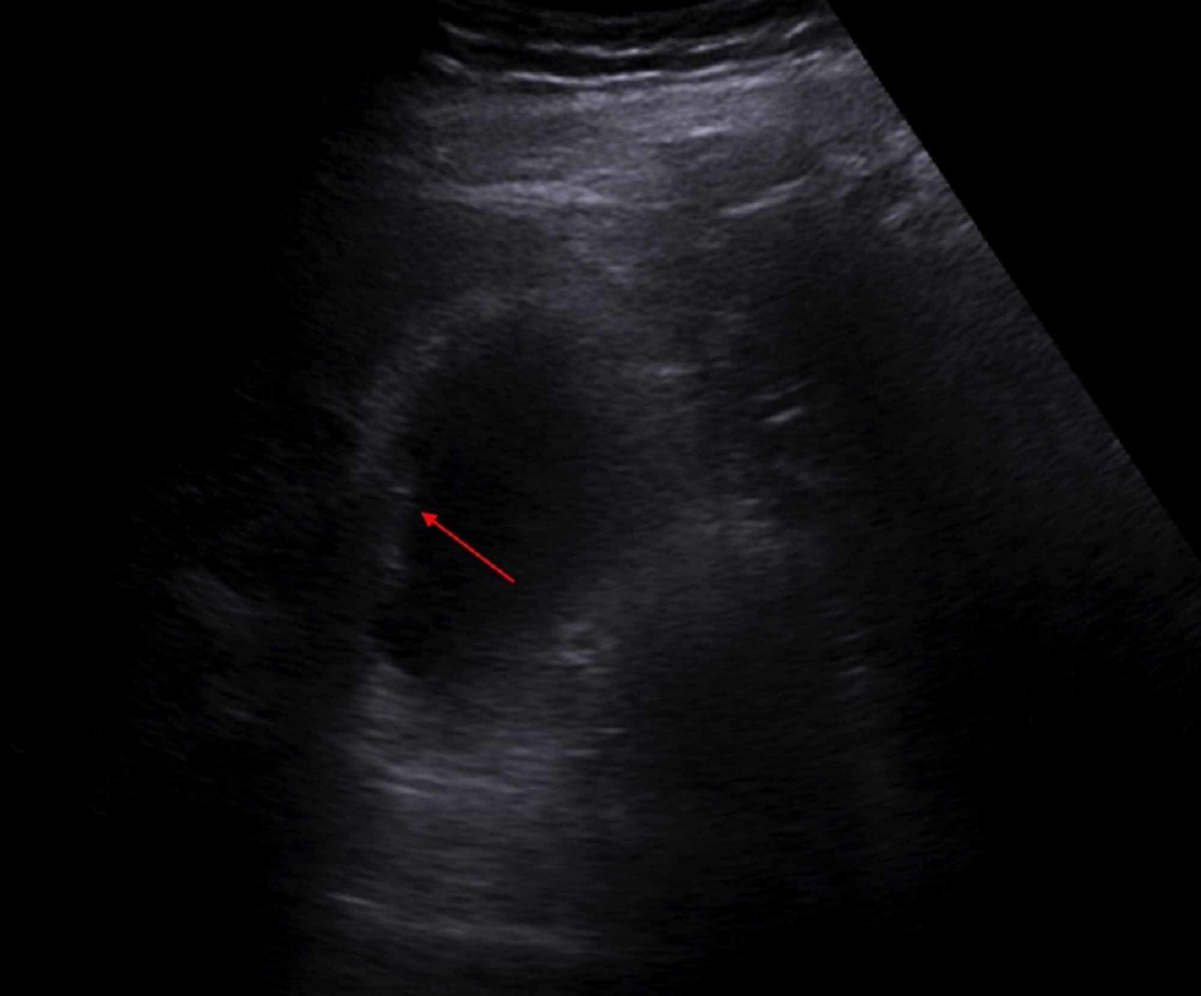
Ultrasound gall bladder showing non-specific, no dependent tissue along the wall (arrow pointing)
